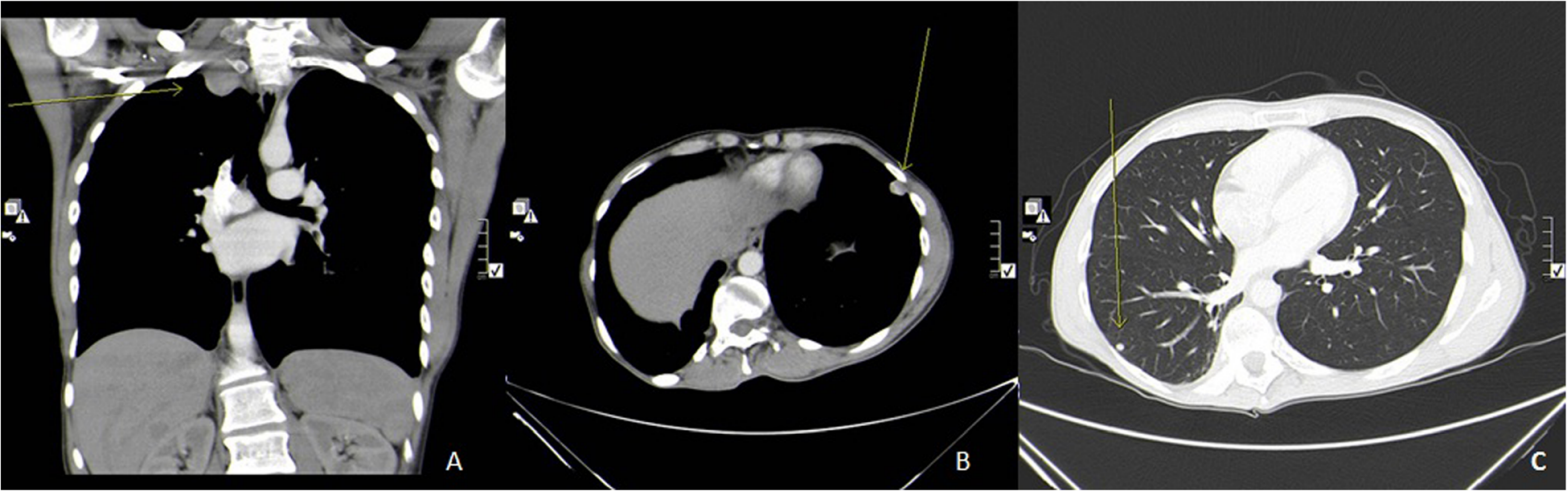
Contrast enhanced chest CT scan. a. Coronal view, mediastinal window. b. Sagittal view, mediastinal window. c. Sagittal view, pulmonary window. Showing three subpleural pulmonary metastasis indicated by yellow arrow
